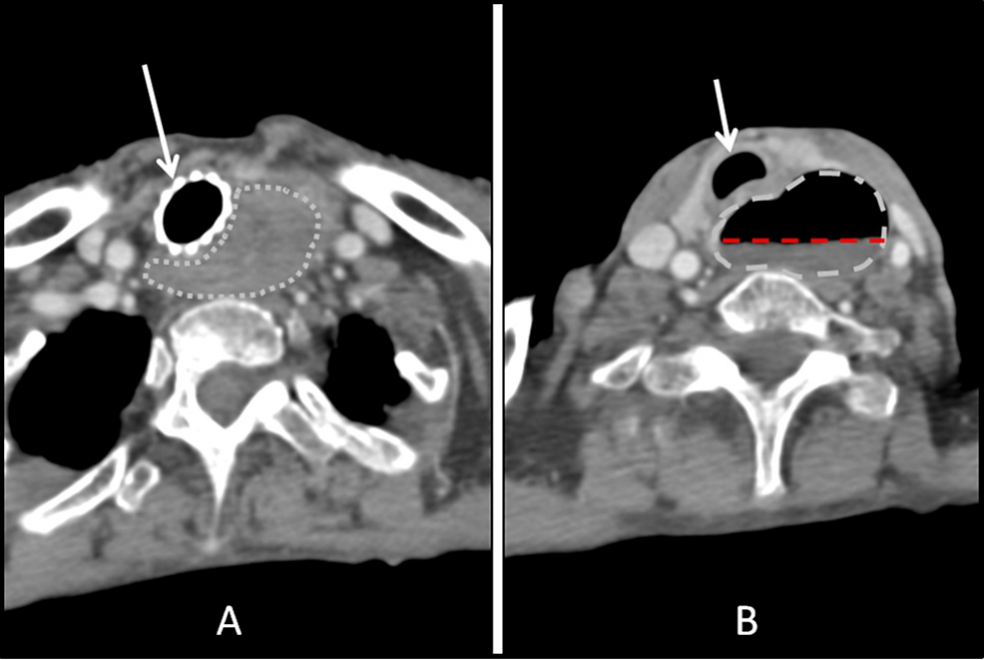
Axial CT scan of the neck and upper thorax with IV contrast performed at baseline A: Axial scan at the level of a seventh cervical vertebra showing bulky esophageal tumor mass (outlined by a gray broken line) without a clear lumen, and trachea with a tracheal stent in place (white arrow). B: Axial scan at the level of a fifth cervical vertebra showing markedly dilated esophagus (outlined by a gray broken line) and containing an internal fluid level indicative of obstruction (red broken line). The trachea is patent at this level (white arrow).
